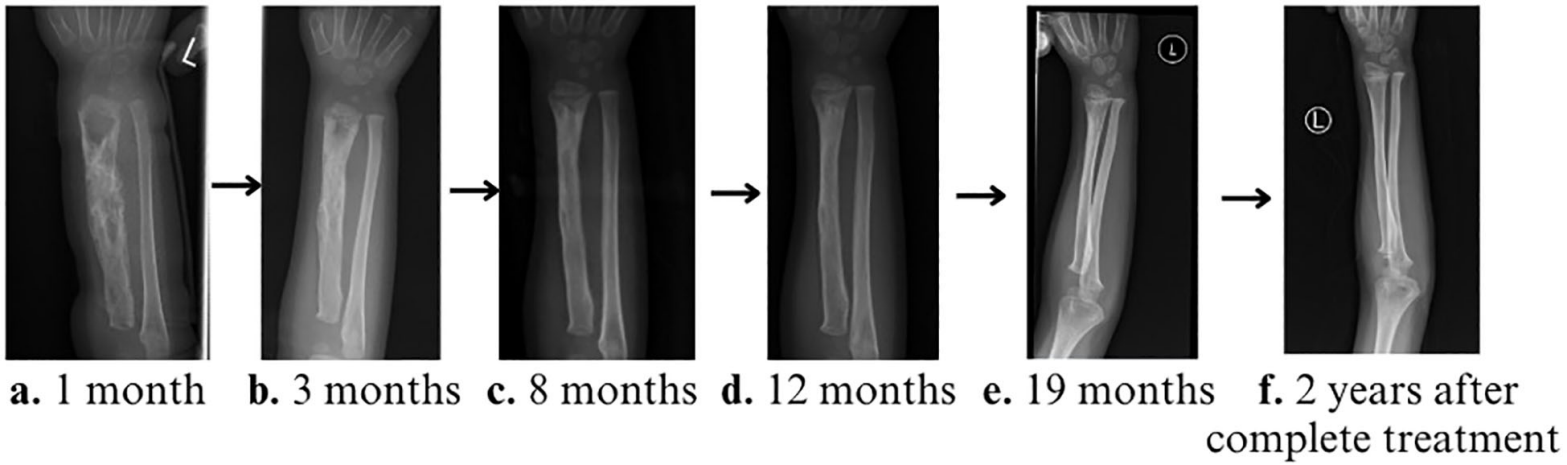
Serial X-rays of left forearm during treatment. (A) 1 month, (B) 3 months, (C) 8 months, (D) 12 months, and (E) 19 months (immediately prior to discontinuation of the anti-tuberculosis medications) showing progressive radiological disease improvement, with near full recovery and remodeling towards the end of the treatment. (F) X-ray of the left forearm 2 years after treatment completion, with full bone remodeling.
